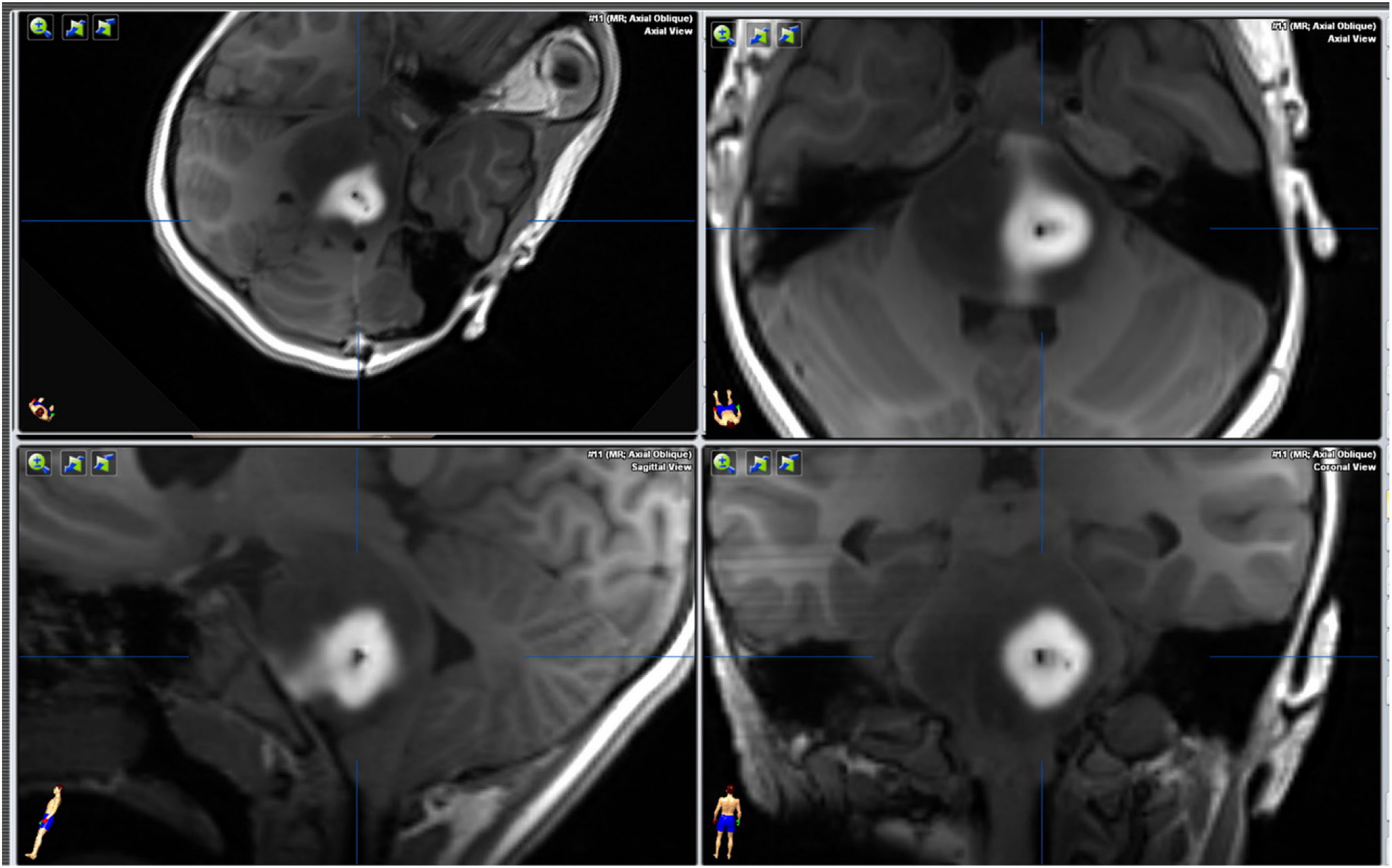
Magnetic resonance imaging T1 GE 3D axial sequence without intravenous contrast, immediately after virus infusion. The intraparenchymal injection of gadolinium before infusing the virus with the MEMS cannula (this cannula has two independent channels) is pushed out from the tumor as the virus is infused from a second channel.
